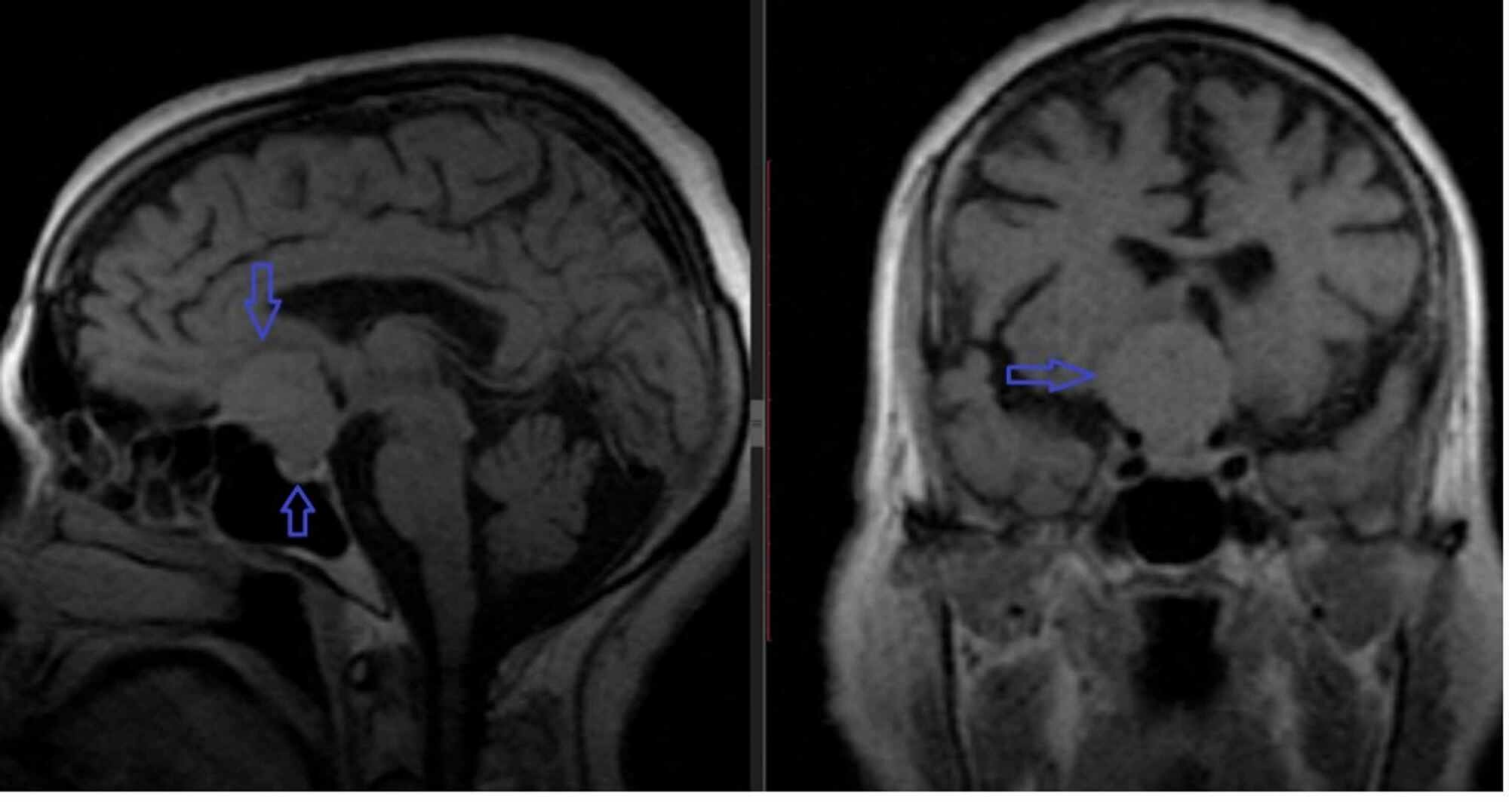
Sagittal and coronal T1-weighted images show a suprasellar mass at the tuberculum sellae, slightly to the right of midline. The mass is compressing the pituitary gland with compression of the optic chiasm superiorly (blue arrows).
